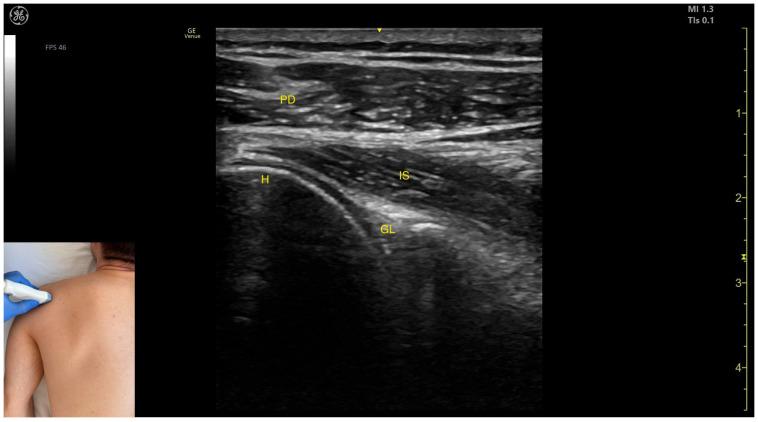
Ultrasound anatomy of the posterior deltoid (PD) with key landmarks: PD—posterior deltoid; IS—infraspinatus; GL—glenoid labrum; and H—humerus.
